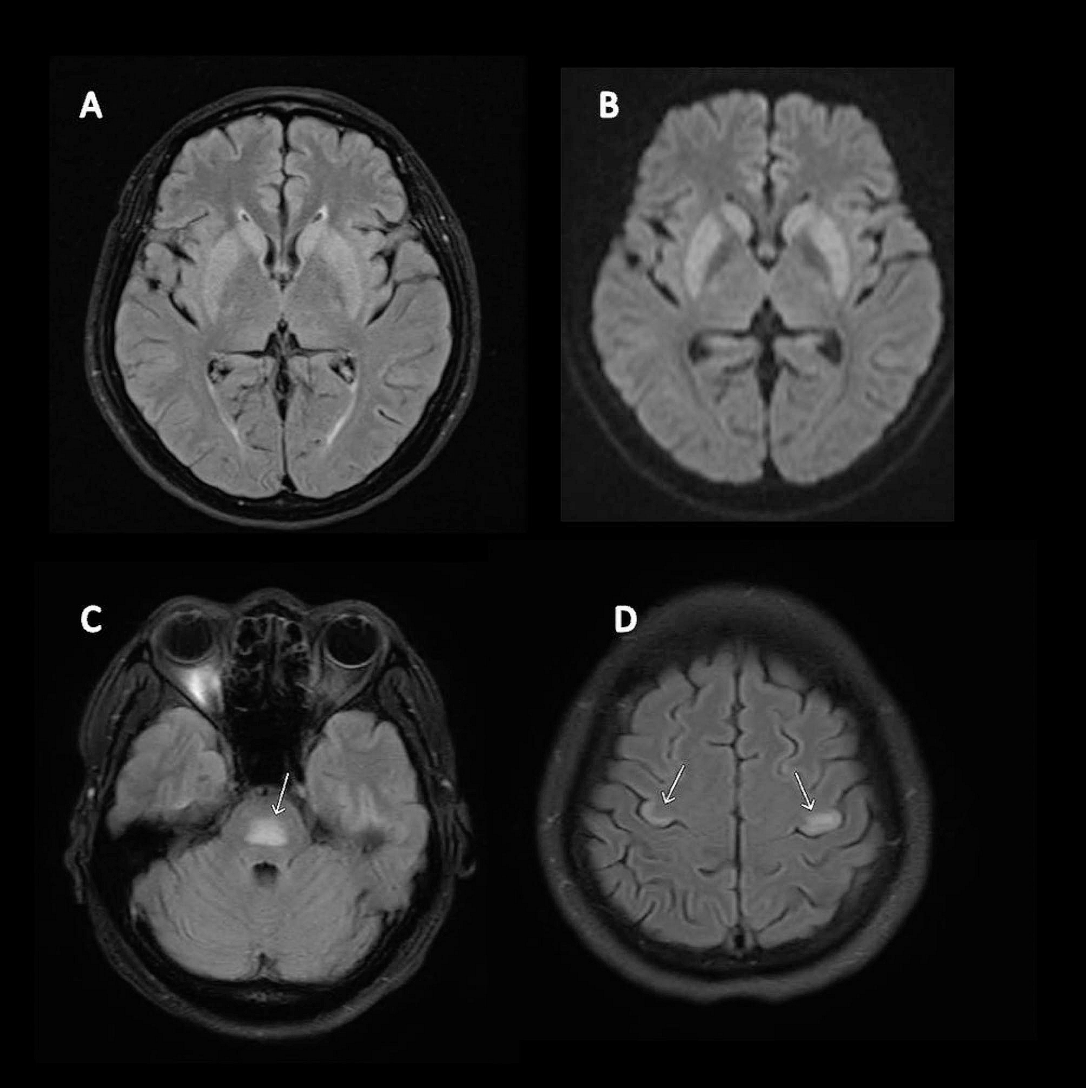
displays magnetic resonance imaging (MRI) of the brain at the onset of symptoms, revealing symmetrical increased signal intensity on FLAIR in the bilateral lentiform and caudate nuclei (A), and hyperintensity on diffusion-weighted imaging (DWI) (B). An MRI of the brain performed three weeks after symptom onset revealed hyperintensity on FLAIR in the pons (C) and bilateral precentral gyri, including the hand knob area (D)
